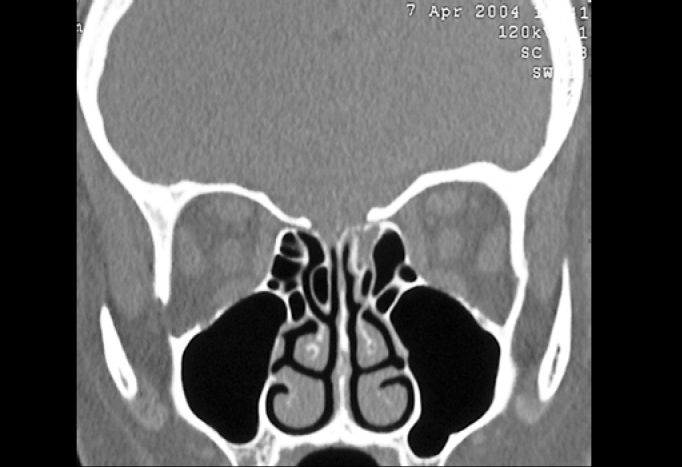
Computed Tomography of patient with CSF fistula in cribriform lamina during left ethmoidal sinusectomy.
